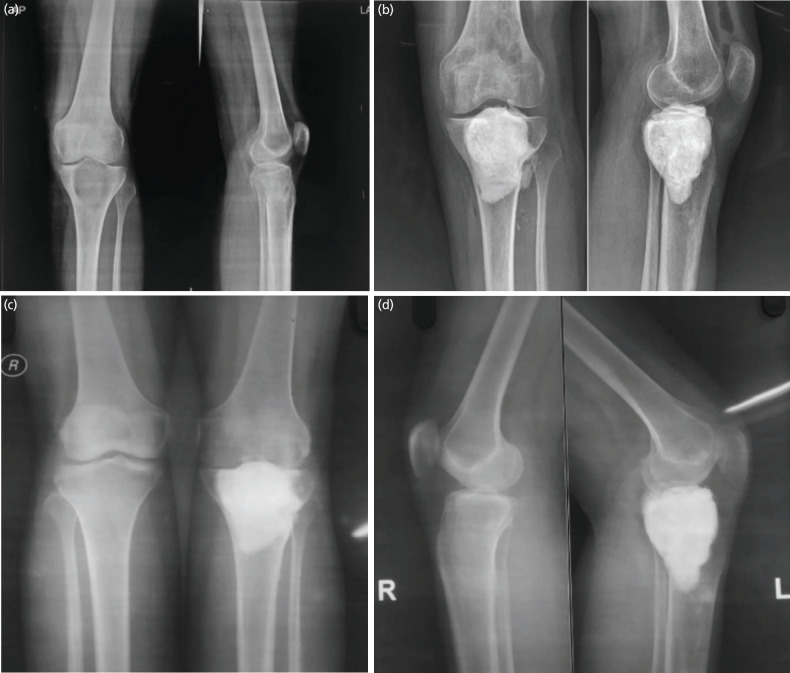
A 27-years old female with Campanacci grade II GCT of left proximal tibia operated with curettage and bone cementation (CBC). (a) Pre-operative radiograph (AP/Lateral) of left knee lytic lesion with expansile lesion having cortical continuity, suggestive of Grade II GCT of left proximal tibia. (b) Immediate post-operative radiograph of patient treated with curettage and bone cementation. (c and d) Radiograph of bilateral knee (AP and Lateral) at final two years follow-up showing well healed lesion with no signs of recurrence of GCT.
